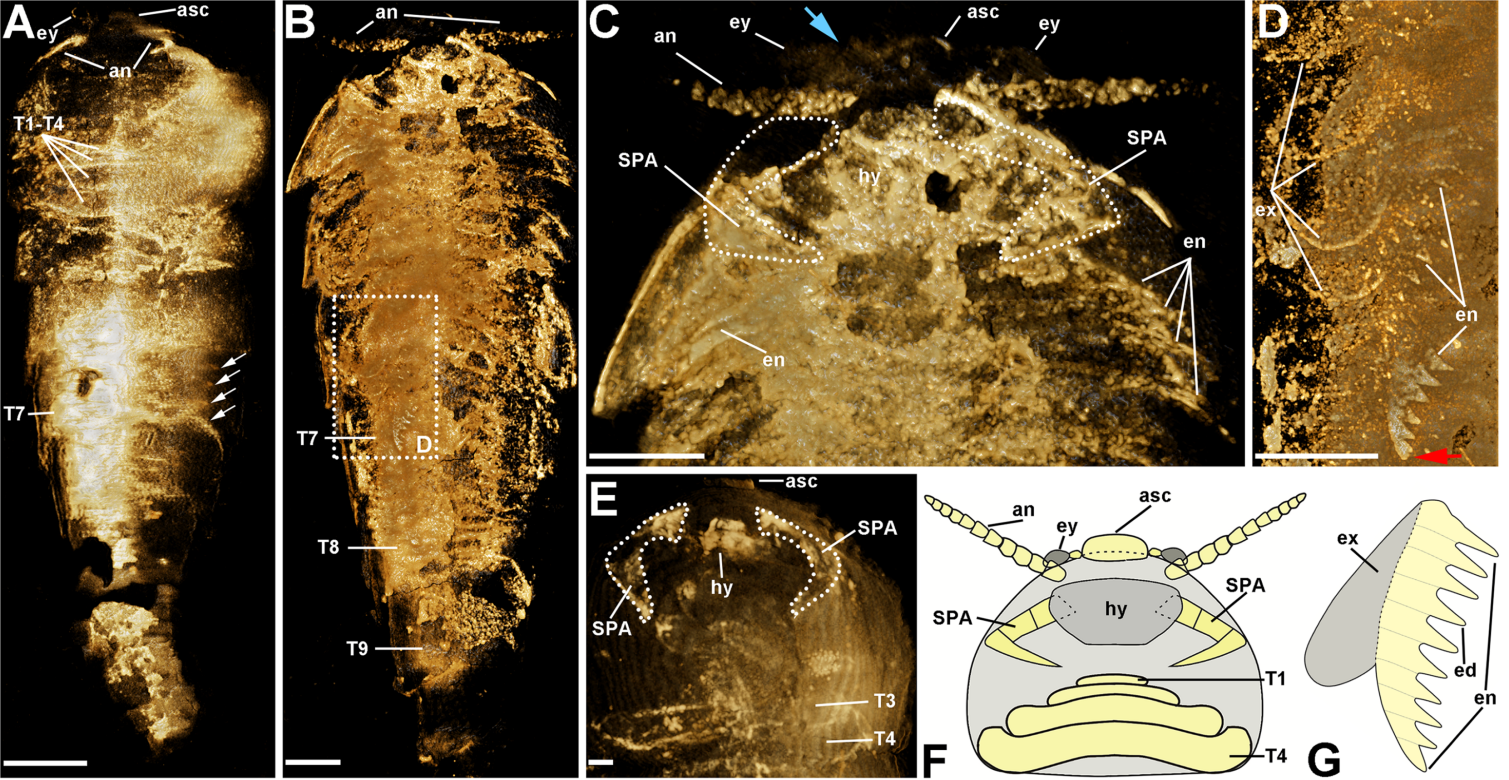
Morphological details of *Pisinnocaris subconigera*. (A) Screenshot of a 3D model derived from a CT scan of the holotype shown in Fig. 1A. Dorsal view showing four anterior shortened segments (T1–T4) and four pairs of appendages (white arrows) associated with T7. (B) Screenshot of a 3D model derived from a CT scan of YKLP 17301 shown in Fig. 1C. Ventral view showing the arrangement of the appendages. Note that there’s no appendage associated with T9 and the subsequent segments. (C) Close-up of the head in B, showing the eyes, the antennae, the SPAs, the hypostome, and the first four pairs of biramous appendages (en). Blue arrowhead indicates the eyestalk. (D) Close-up of the trunk in B, showing four flap-like exopods (ex) preserved on T6, along with an almost complete endopod in T7 (en). Red arrowhead indicates the distal podomere of the endopod in subtriangular shape. (E) Screenshot of a 3D model derived from a CT scan of CJHMD00066a shown in Fig. 1D. Ventral view showing a pair of SPAs with the hypostome (hy) in-between. The last two shortened prothoracic segments (T3, T4) are also detected. (F) Reconstruction of the ventral side of the head. (G) Reconstruction of a biramous appendage consisting of a flap-like exopo d (gray) and an endopod bearing at least ten podomeres (yellow). Abbreviations: an, antenna; asc, anterior sclerite; ca, carapace; ed, endite; en, endopod; ex, exopod; ey, eye; hy, hypostome; SPAs, specialized post-antennal appendages; T*n*, trunk segment *n*. Scale bars: 1 mm.
